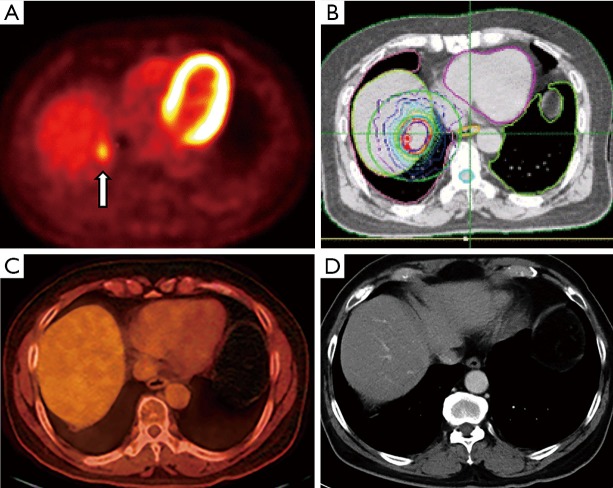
A 61-year-old male with history of localized rectal cancer treated with chemoradiation and surgery; subsequently diagnosed 2 years later with isolated liver lesion growing in size and avidity, biopsied positive for metastatic rectal cancer. Patient initially refused all invasive procedures including surgery, opting for chemotherapy alone, then ultimately agreed to SBRT. (A) Pretreatment axial fused PET-CT image demonstrating metastatic segment 7 liver lesion with SUV 3.8 (arrow). (B) Treatment plan depicting prescription isodose line (in red) with rapid dose fall off around the tumor. (C) Axial fused PET-CT obtained 18 months after SBRT demonstrating no evidence of disease. (D) Axial contrast enhanced CT image obtained 4 years after SBRT demonstrating no evidence of disease. SBRT, stereotactic body radiation therapy.
